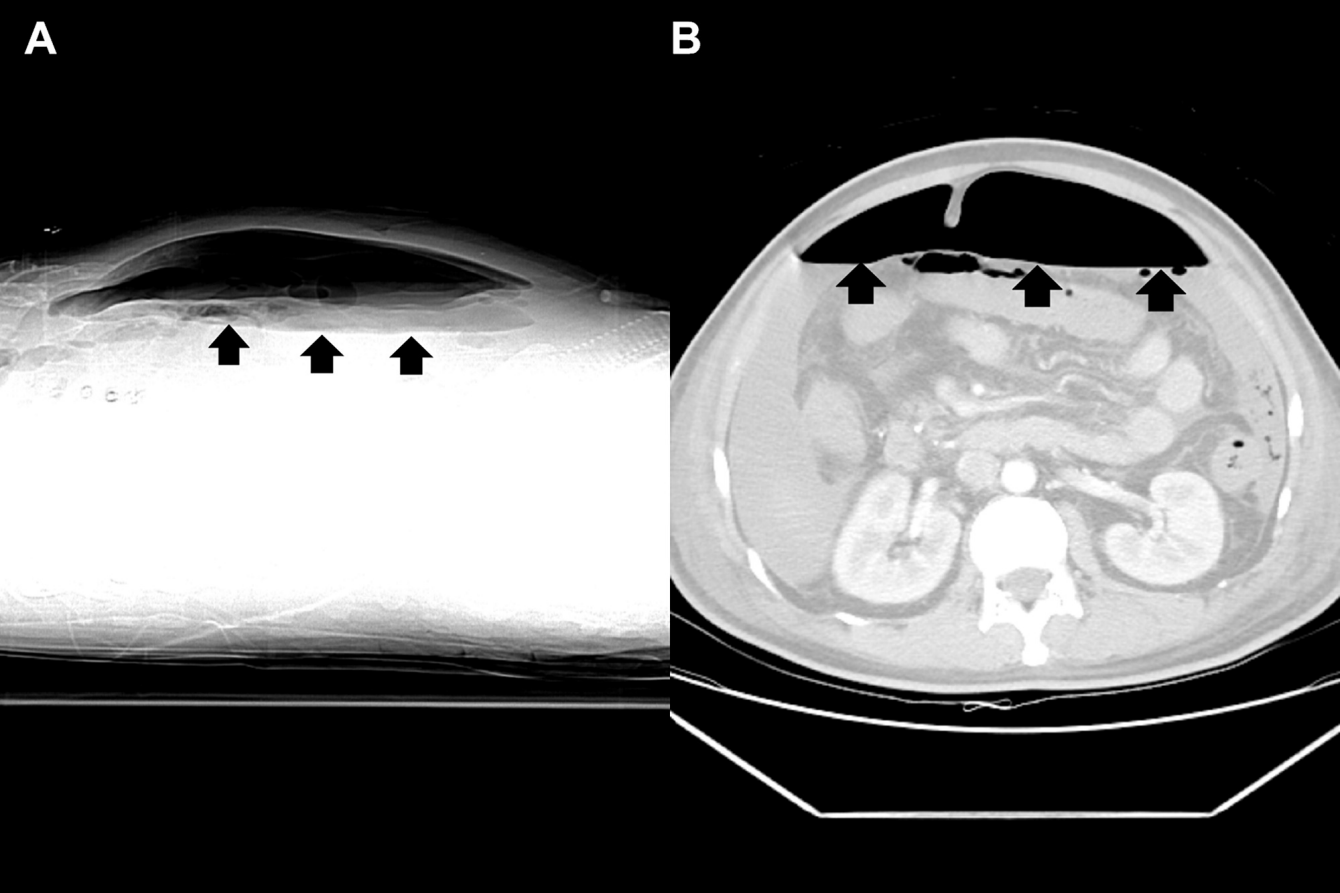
Intraperitoneal free air (black arrows) on abdominal computed tomography
